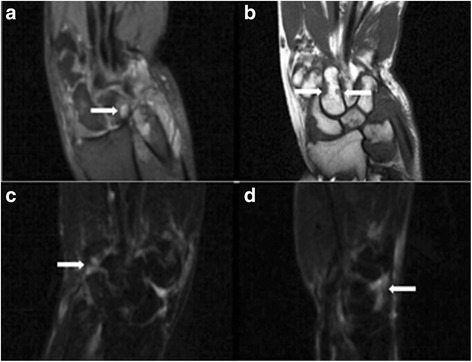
Typical case: 52-year-old female diagnosed with RA for 1 year. MRI: carpal synovitis with bone destruction. a T2WI: oval high signal within the lunare bone—pannus formation. b T1WI: carpal bones showed low signal loss—bone destruction. c, d T2WI: carpal synovitis
